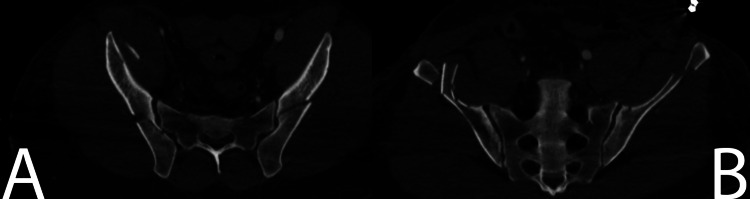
Reformatted CT images of the posterior pelvic ring. (A) Inlet reformatted CT image of the posterior pelvic ring demonstrating bilateral fracture lines entering the sacroiliac joint. (B) Outlet reformatted CT image of the posterior pelvic ring demonstrating diastasis of the upper sacroiliac joint consistent with crescent fracture-dislocation. CT: computed tomography
